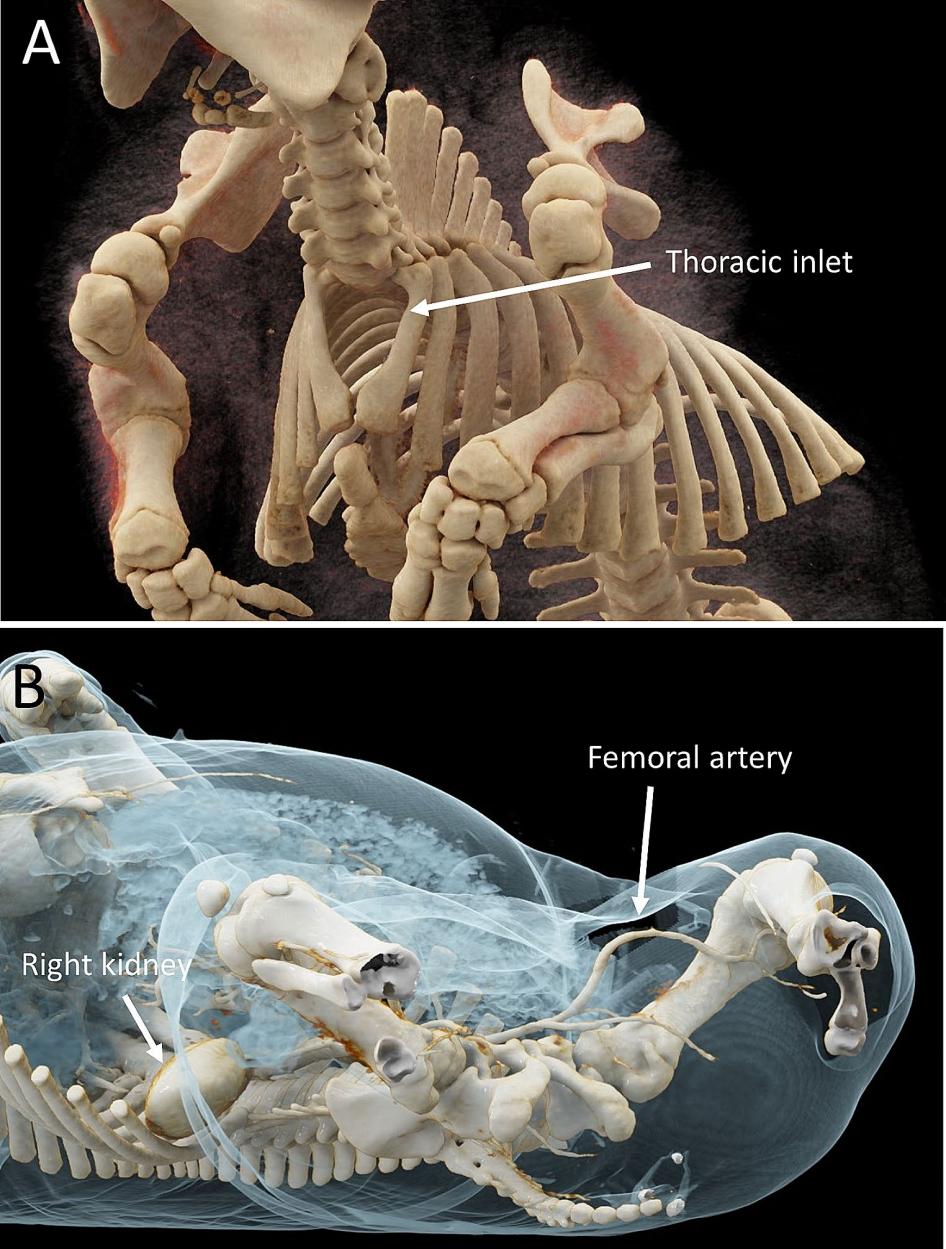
3D renderings of computed tomography showing (A) the configuration of the neck and thoracic cage. (B) femoral artery exiting the pelvis and point of access in the hind leg
